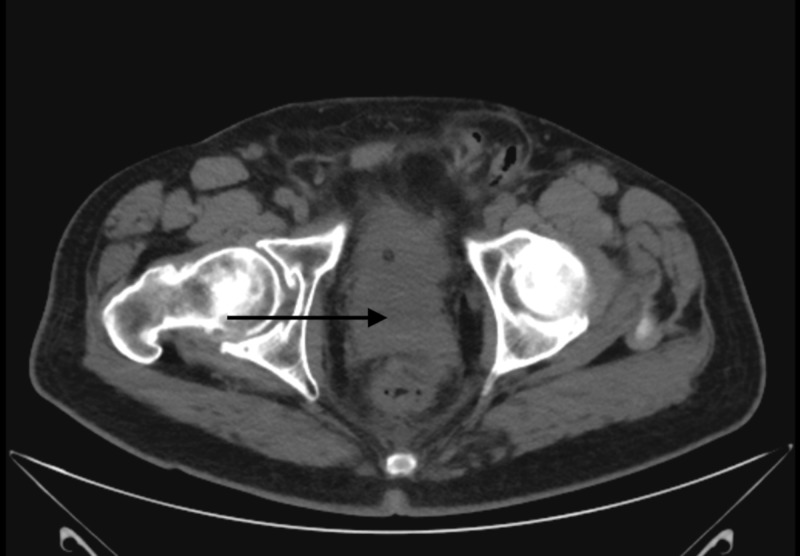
Axial view of the CT pelvis Black arrow points toward the prostate
